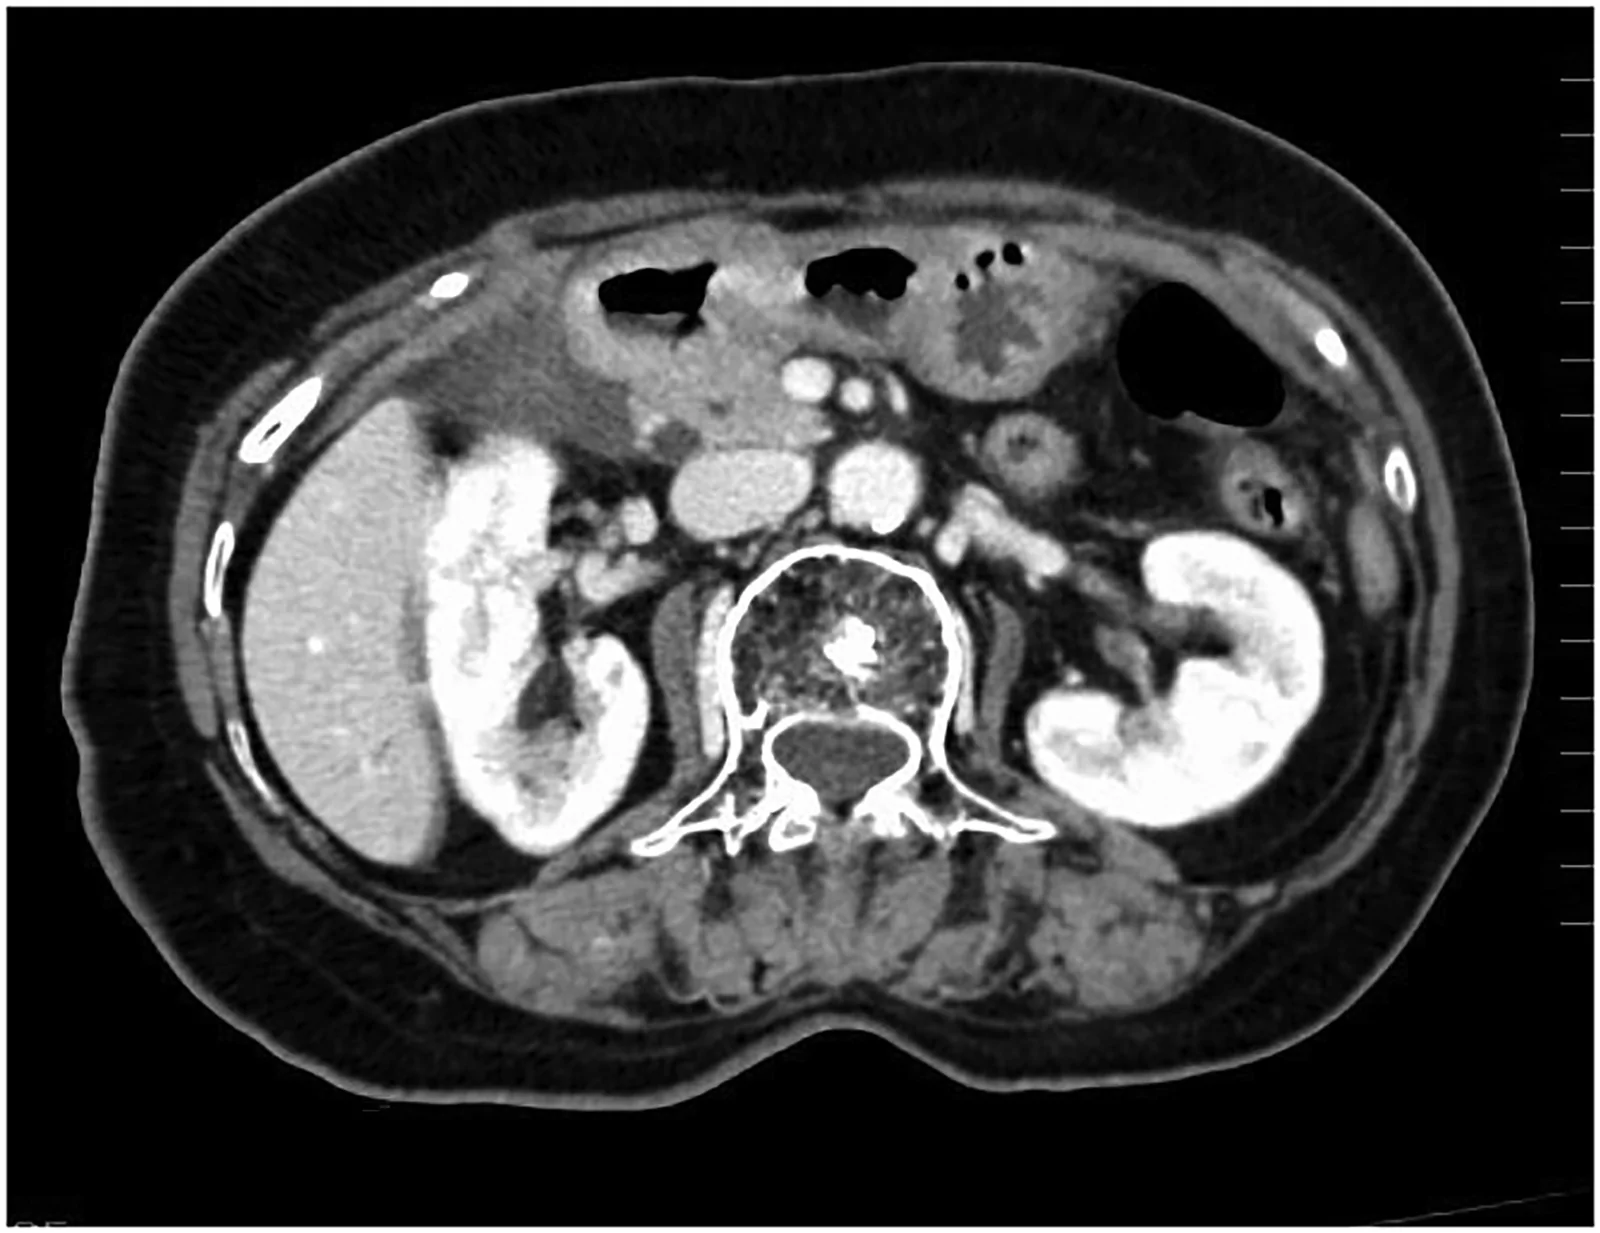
CT finding at admission showed mild gallbladder wall thickening, slight effusion around the gallbladder, and a lack of ascites.
